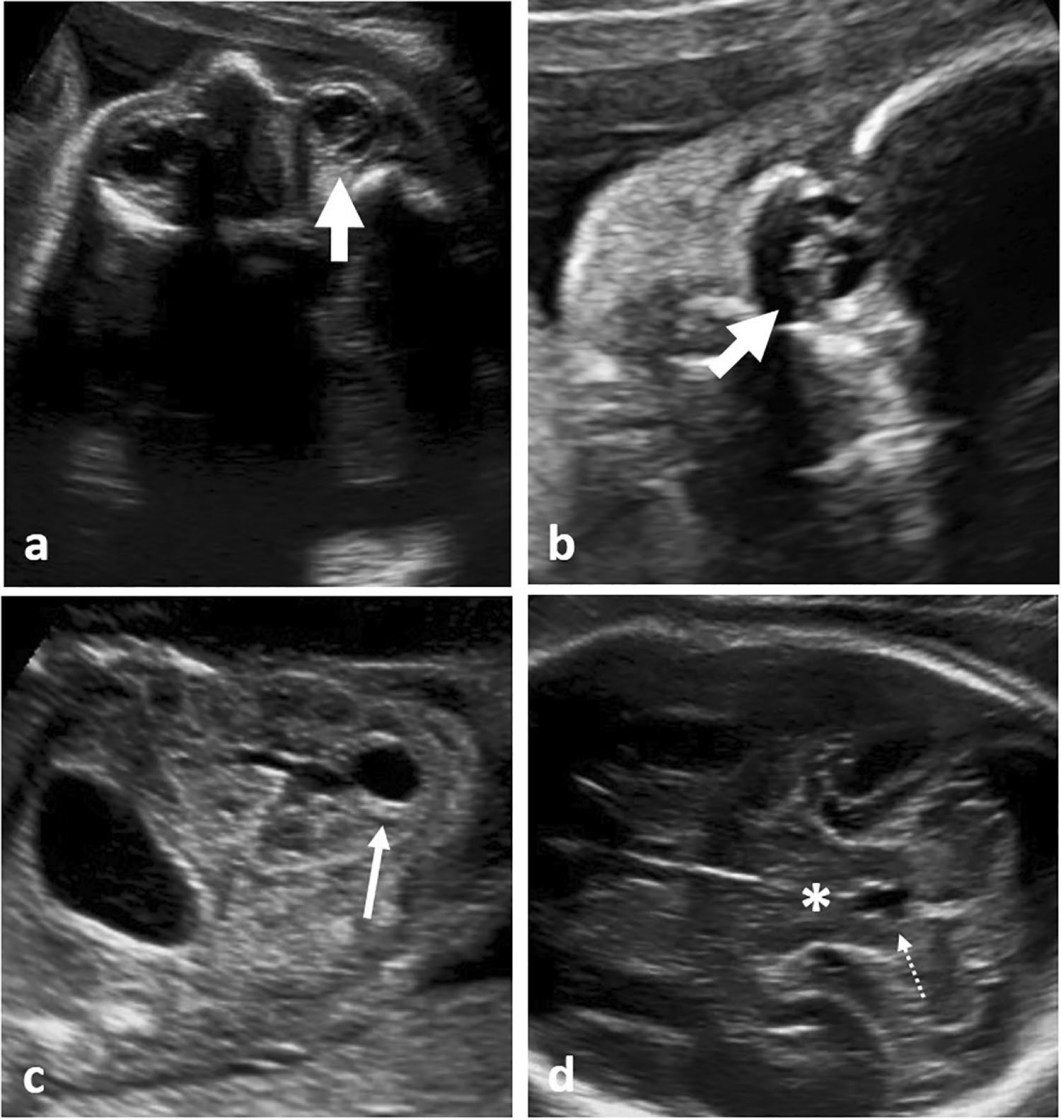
Prenatal ultrasound in a fetus with Joubert syndrome at 25 + 3 weeks; a bilateral microophthalmia with unilateral ocular coloboma (thick, short white arrow); b ocular coloboma (thick, short white arrow); c unilateral kidney cyst (thin white arrow), otherwise normal kidney structure; d molar tooth sign (*), note the elongated appearance of the fourth ventricle (thin, dotted white arrow)
